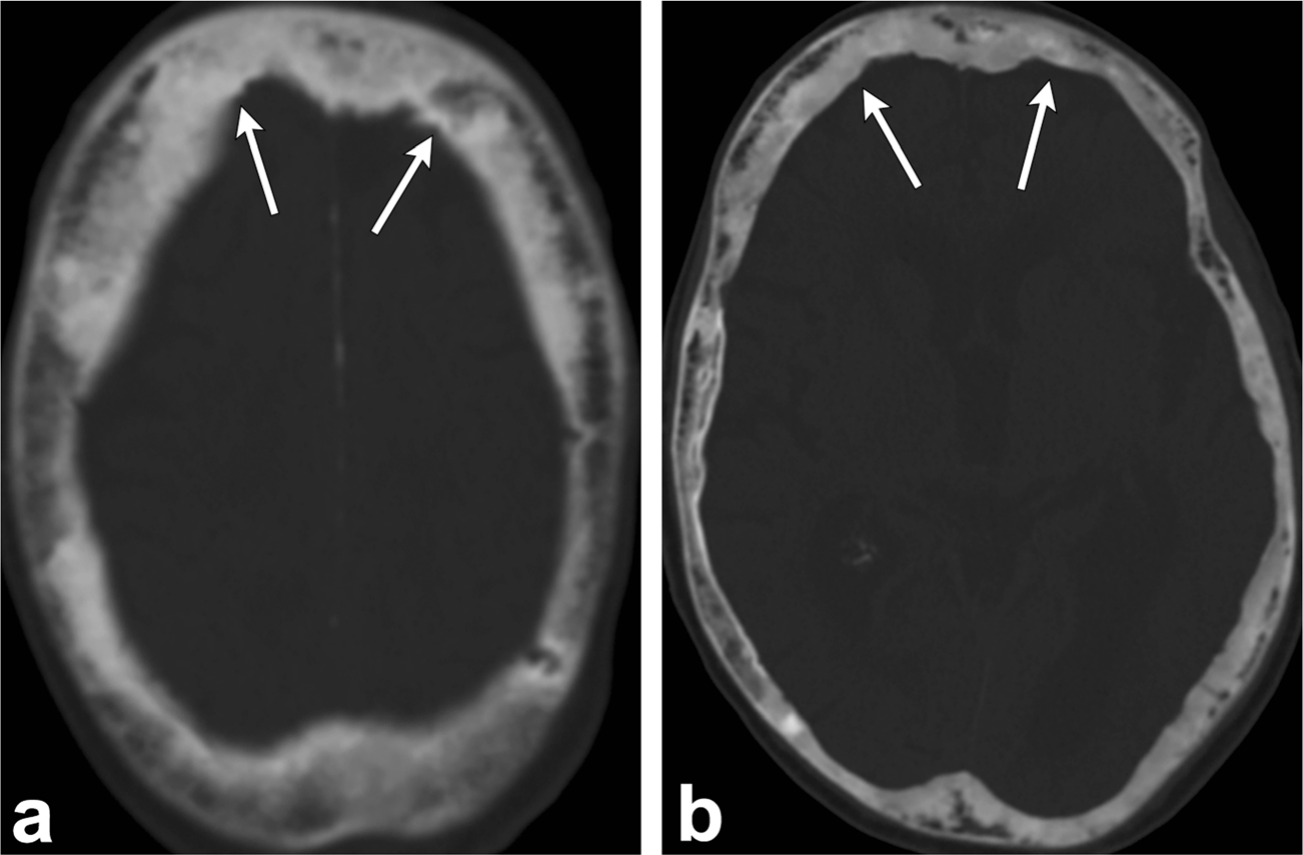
Paget disease. Axial head CT (a, b) images demonstrate increased cortical density and trabecular thickening (*arrows*)
